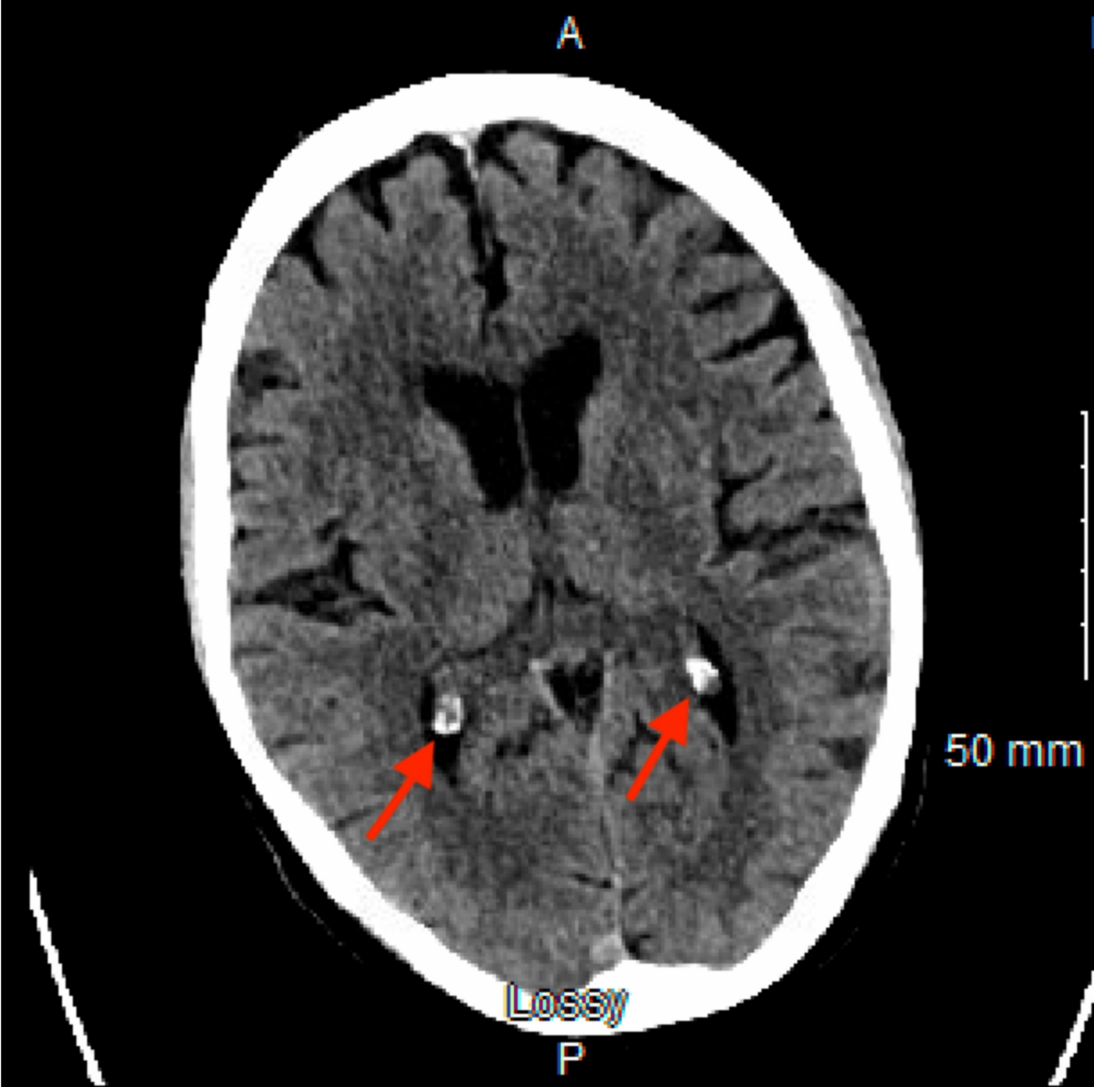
Unremarkable head CT without contrast Red arrows indicate calcification of the choroid plexus (normal aging process).
